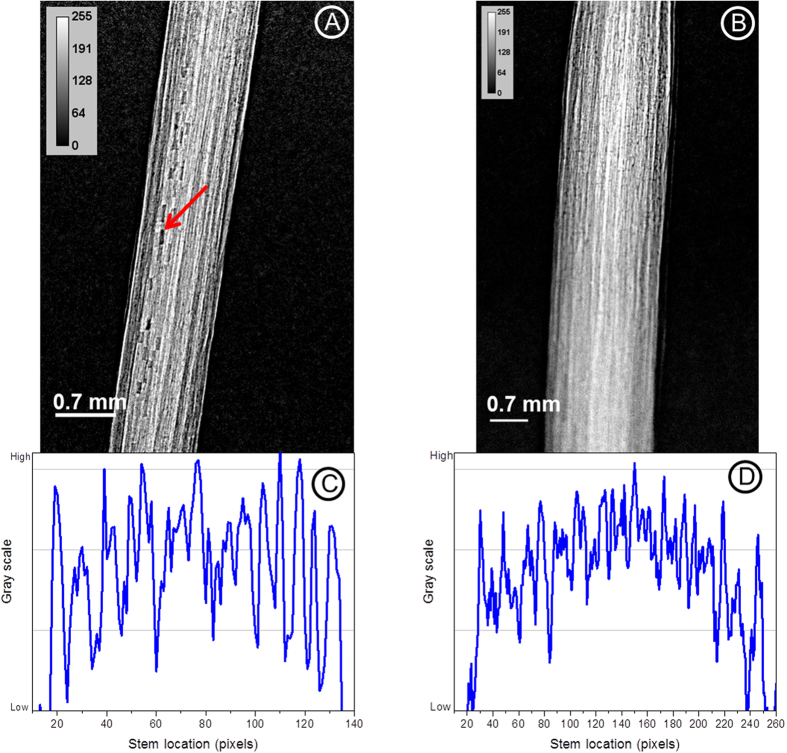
Effect of effective pixel size on plant imaging. X-ray images of a canola stem recorded at 18.0 keV using detectors with an effective pixel size of 8.75 μm (A) and 4.3 μm (B). Line profiles (C,D) across the centre of the stem from (A,B). Arrow indicates stem cavitation.
